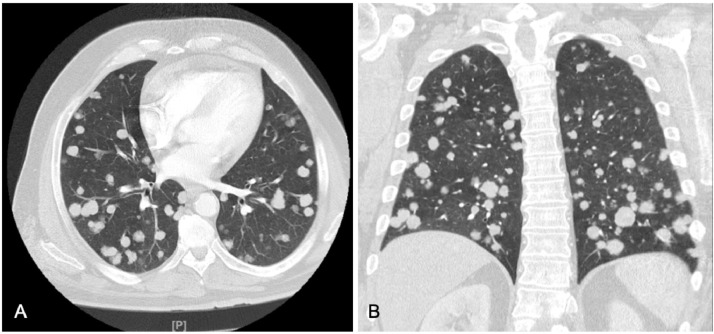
Axial (A) and coronal (B) lung window images from a CT with contrast demonstrating multiple bilateral round pulmonary metastasis.
